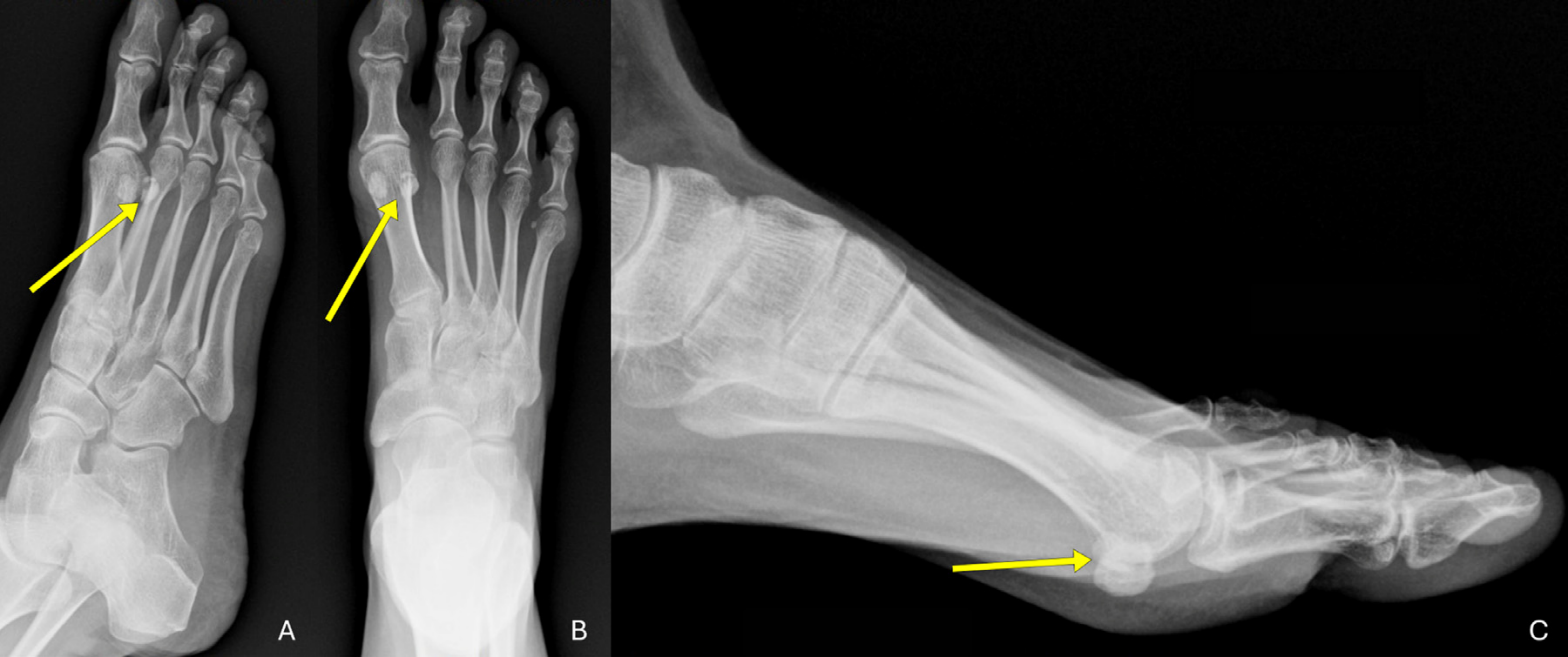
(A) Anteroposterior (AP), (B) oblique, and (C) lateral radiographs of the left foot. Yellow arrows indicate sclerosis and fragmentation of the lateral hallucial sesamoid, suggestive of AVN.
